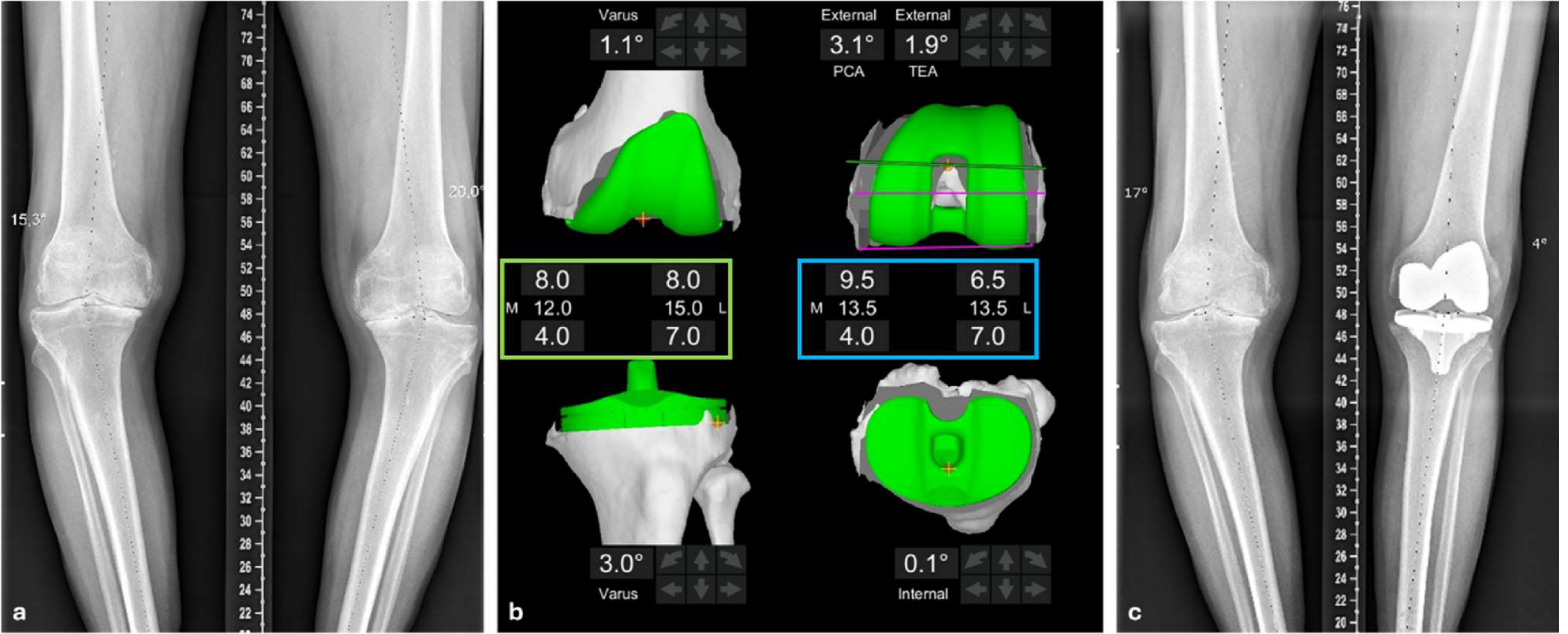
Management of a patient with a bilateral knee osteoarthritis with large deformity. a: preoperative long leg standing X-rays showing a varus deformation of 20°. (b) Intra-operative Mako settings. Coronal femoral component positioning is set to restore the native joint surface. Coronal tibial component positioning is set to obtain an equal medial and lateral extension gap. The green box represents the value of the distal femoral cuts (values at the top of the box), and the proximal tibia cuts (values at the bottom of the box). The middle values represent the total cut thickness. For this case, femoral varus is set at 1.1° and tibial varus is set at 3° to achieve this goal. In the axial plane, femoral component is positioned to align with the trochlear groove while having an equal medial and lateral flexion gap. The blue box represents the thickness of the posterior femoral cuts (values at the top of the box) and the proximal tibial cuts (values at the bottom of the box). The middle values represent the total cut thickness. For this case, femoral component rotation is set at 3.1° relative to the PCA (Posterior Condyle Axis) or 1.9° relative to the sTEA (surgical Trans-Epicondylar Axis). (c) One-year postoperative long-leg standing X-rays showing a global varus value of 4°.
